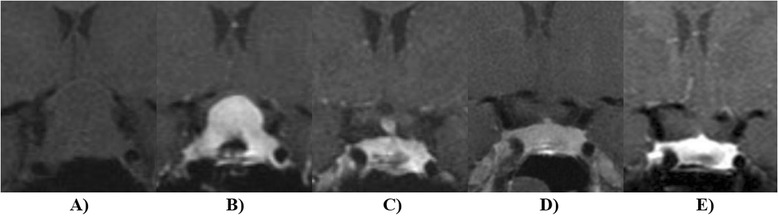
Hypophysitis was diagnosed in a 30 year old during the late third trimester of pregnancy. The patient presented with 3 weeks of progression vision loss. Panel a depicts a coronal pre-contrast T1-weighted image of the pituitary. Transsphenoidal biopsy (Panel b) demonstrated lymphocytic hypophysitis and glucocorticoid therapy was begun with prednisone 60 mg daily. Following delivery, the pituitary gland decreased in size (Panel c) and remained stable 2 months (Panel d) and 5 months (Panel e) after glucocorticoid taper and discontinuation
